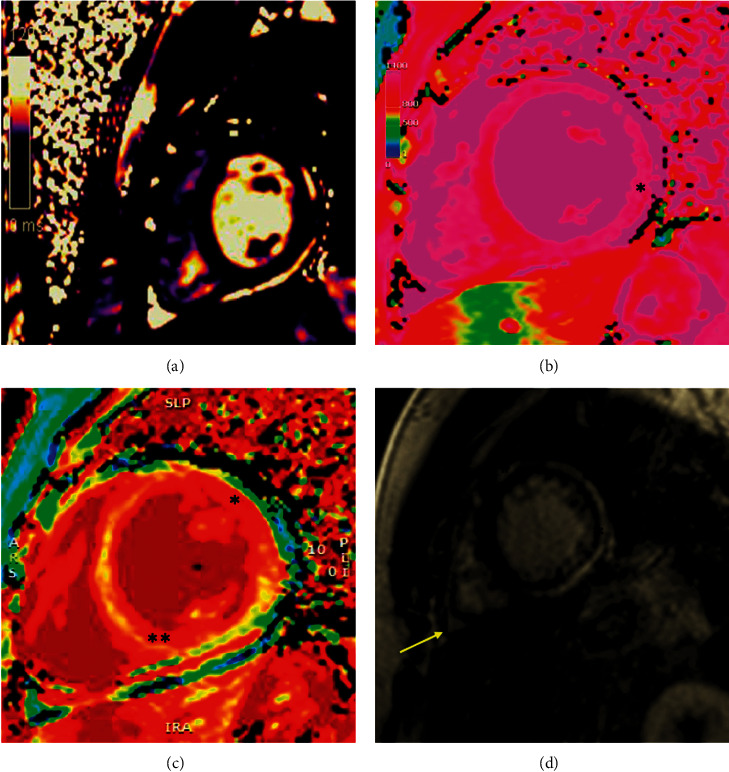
Parametric mapping and tissue characterization in myocarditis on cardiac MRI. (a) Short-axis midmyocardial T2 map with normal relaxation time and no evidence of acute edema. (b) Precontrast native midmyocardial T1 map showing diffusely elevated T1 relaxation time specifically along the lateral wall (^*∗*^). (c) Midmyocardial extracellular volume (ECV) map indicating increased ECV along the anterior and lateral walls (^*∗*^) and the inferoseptal wall (^*∗∗*^). (d) Postcontrast pulse sequence inversion recovery image of the midmyocardium with increased pericardial signal (^*∗∗*^), evidence of diffuse subepicardial late gadolinium enhancement along the entirety of the lateral wall (^*∗*^), and mild pericardial effusion (arrow).
